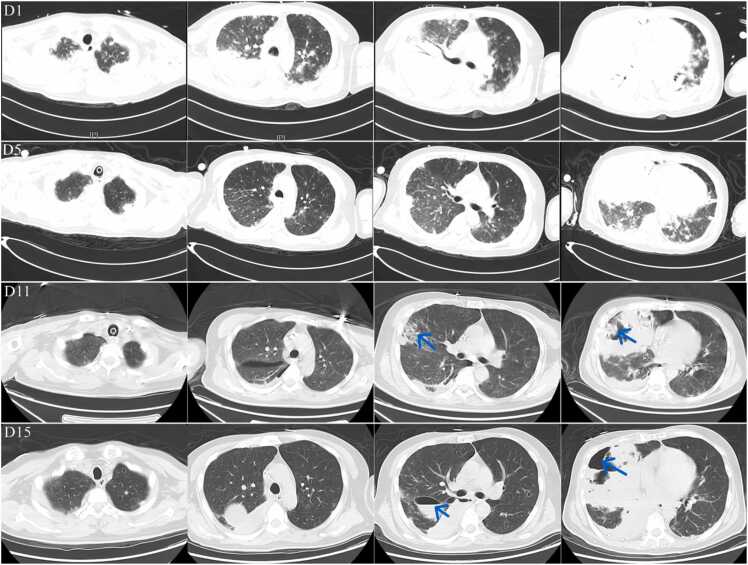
Progression of Pulmonary Findings on Serial Computed Tomography (CT). These representative axial CT images, obtained on days 1, 5, 11, and 15 after admission, are displayed in chronological rows. Each column corresponds to a consistent anatomical level, allowing for direct comparison of pathological changes in the upper, middle, and lower lung zones over time. D1 (top row): Extensive, diffusely distributed ground-glass opacities and consolidations are observed in both lungs, partially coalescing into a “white lung” appearance, most prominent in the posterior and basal regions. These findings are consistent with the radiological features of very severe ARDS. D5 (second row): After initiation of VV-ECMO and antimicrobial therapy, there was partial resolution of the diffuse infiltrates with some improvement in aeration, though extensive opacities and consolidations persisted. D11 (third row): While most infiltrates continued to clear, the improvement was asymmetric. A new cavitary lesion with thick walls, an air–fluid level, and surrounding consolidation emerged in the right middle and lower lobes (arrow), a finding highly suggestive of a developing lung abscess. D15 (bottom row): After percutaneous drainage of the abscess under CT guidance, the cavity was substantially smaller, with thinner walls and less surrounding inflammatory infiltration. The continued resolution of other pulmonary opacities signified marked radiological improvement.
